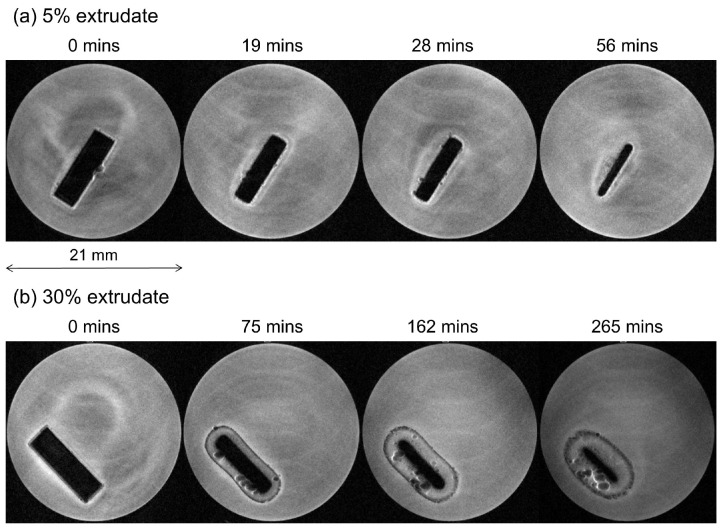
Magnetic resonance images (first experiment) showing the changes as a function of time in one cross sectional slice of the extrudates containing 5% (a) and 30% (b) of bicalutamide.
